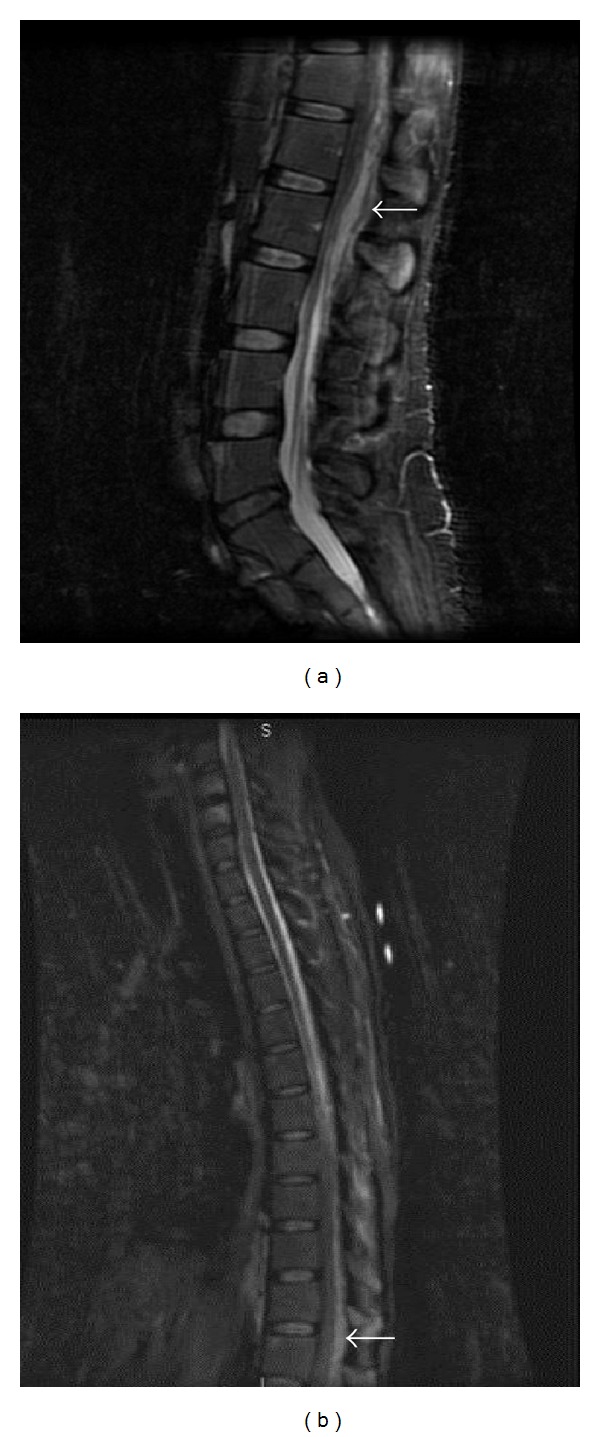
MRI of the lumbosacral spine (panel (a)) and thoracic spine (panel (b)), sagittal view: complex posterior epidural collection extending from lumbar L4 level cephalad into thoracic T7 associated central canal compromise and findings consistent with epidural abscess.
